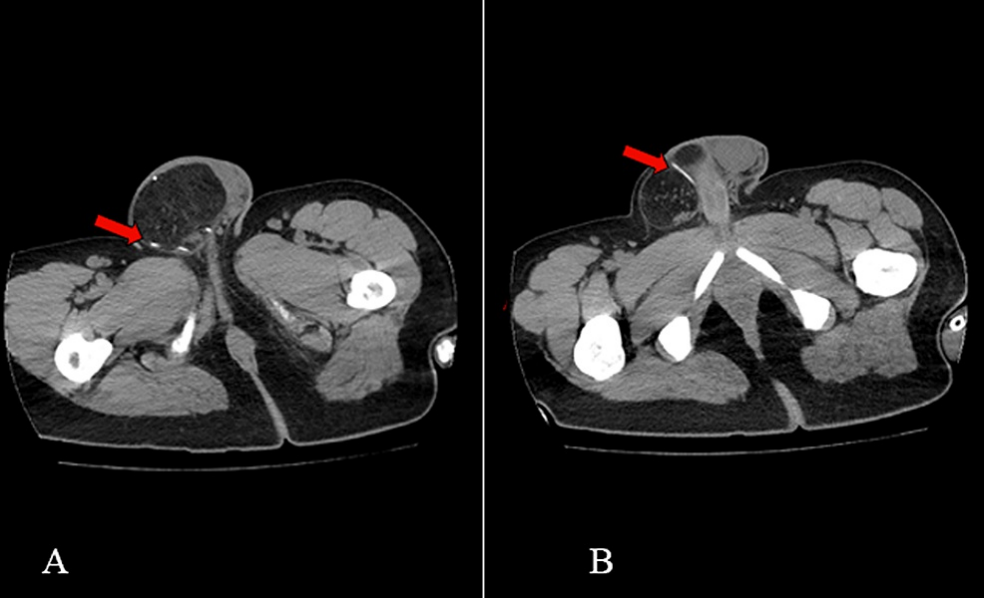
Migration of abdominal catheter Axial sections of abdominal CT scan showing hyperdensity (red arrows) corresponding to a coiled catheter (A) and a straight catheter (B) inside the scrotum, having migrated with the inguinal hernia.
